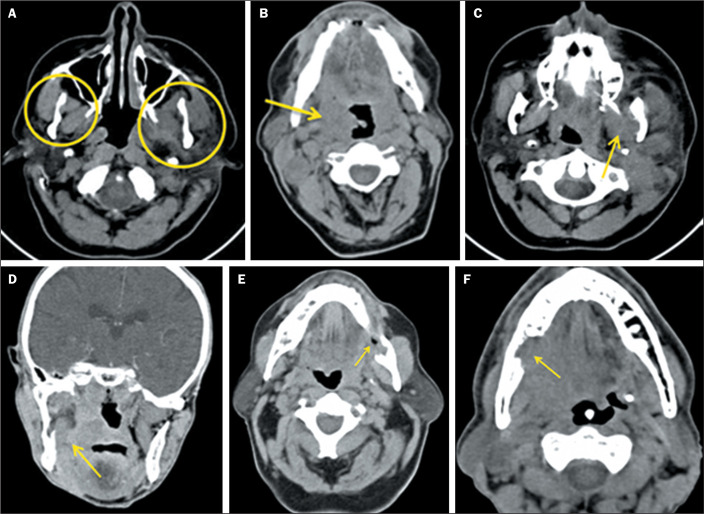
A-C: Axial CT slices with soft-tissue window settings, demonstrating
